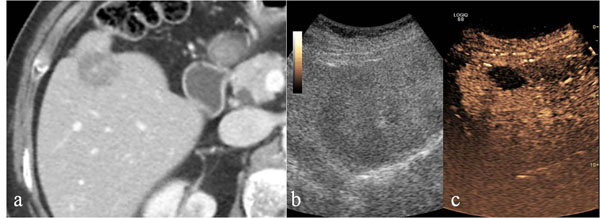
Liver. Contrast-enhanced CT in venous phase (a), US-B-mode (b) and CEUS (c) in a 67 year-old male patient with blunt abdominal trauma. CT shows a epatic hypodense intra-parenchymal traumatic area (a) not recocognizable by US B-Mode examination (b). CEUS demonstrate epatic hypoechoic lesion corresponding to that of CT.
